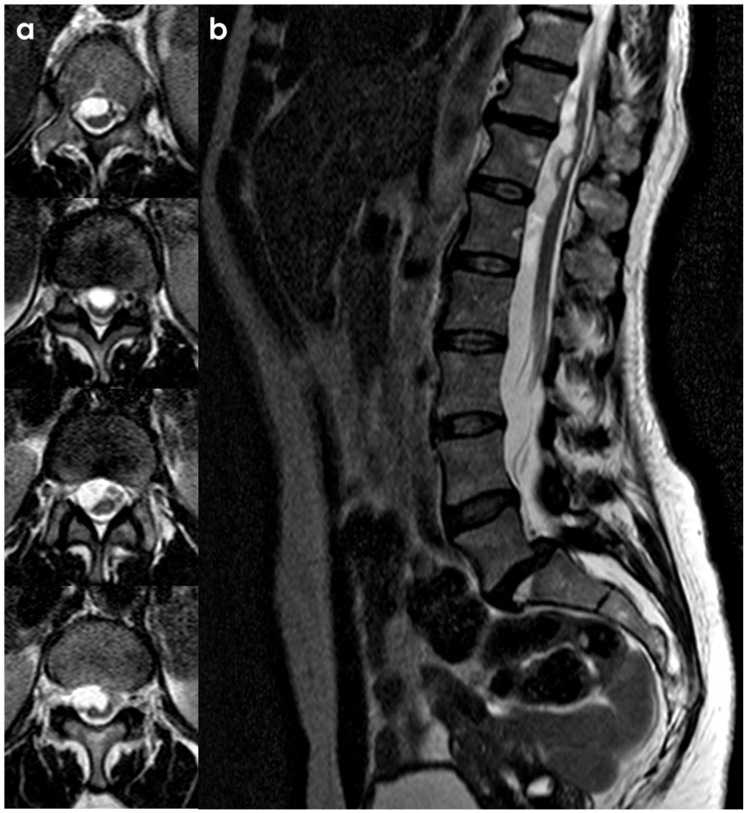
Spinal multiloculated arachnoid cysts with deformation and dislocation of the spinal cord in axial (a) and sagittal (b) T2W MRI.
